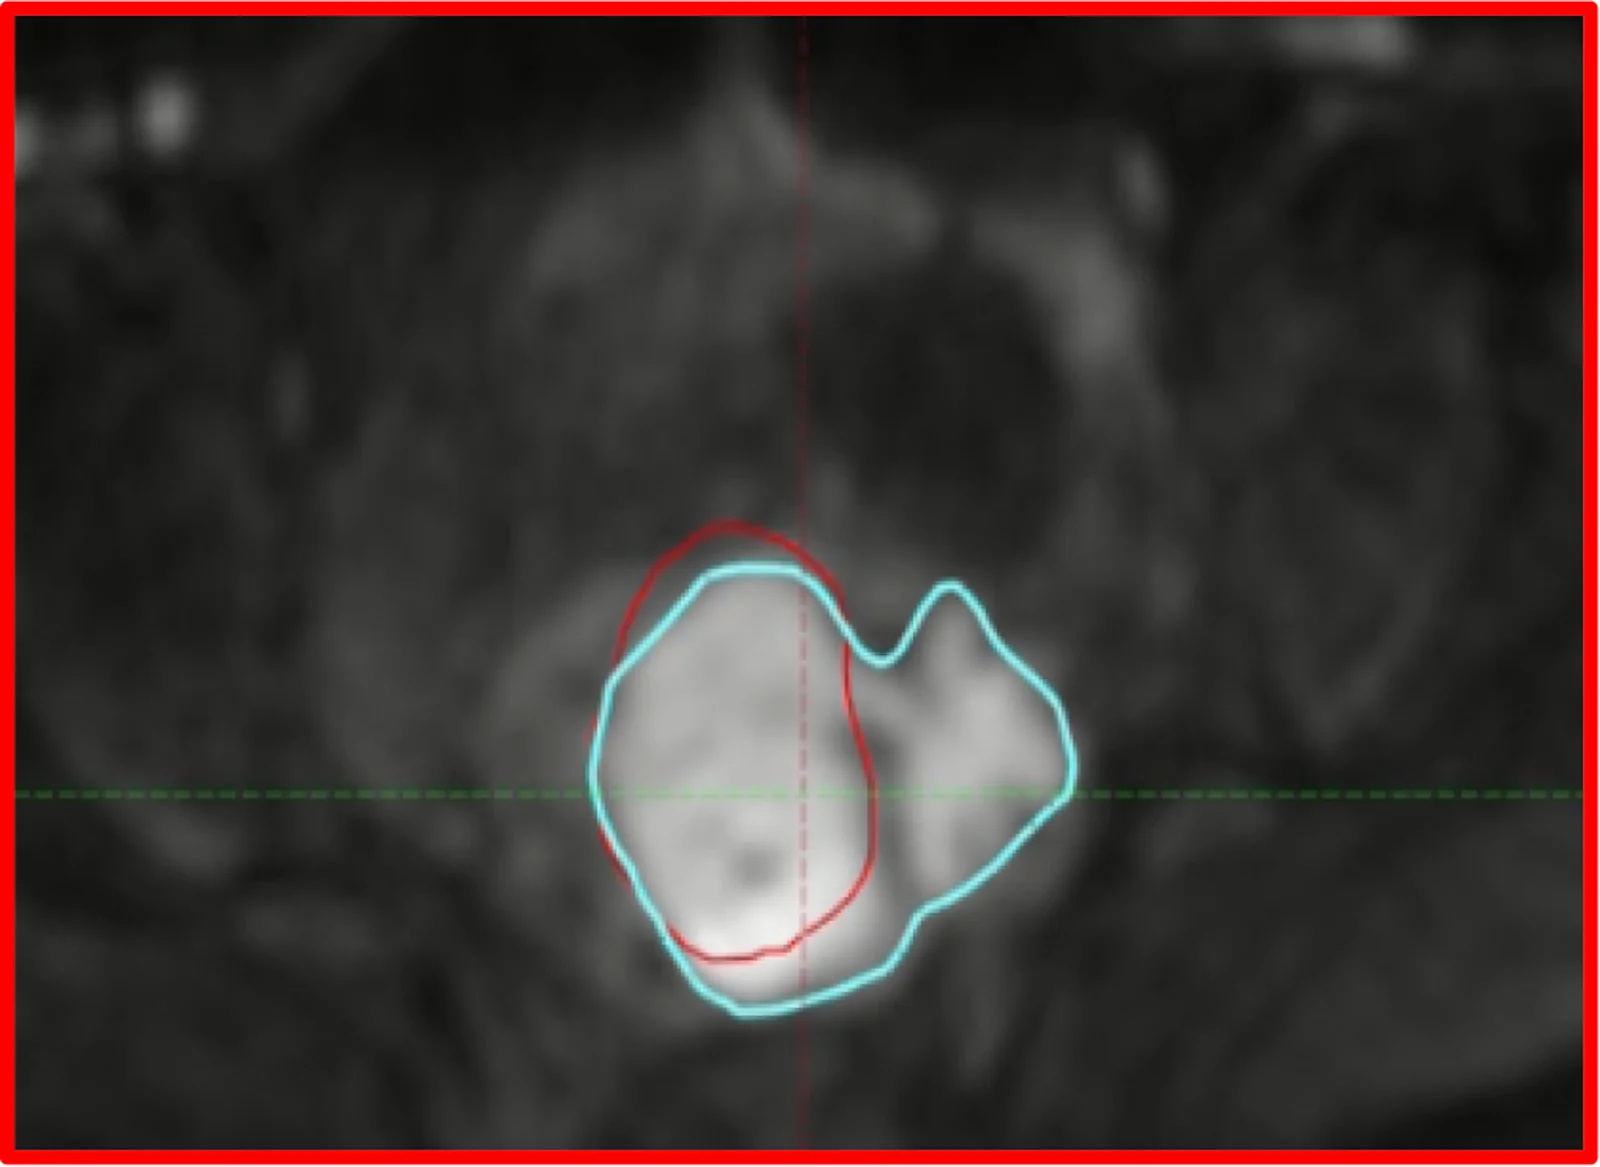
a. Figure depicting the GTV at pre_EBRT, GTV_T2 in red outline with an adjacent hypointense mass in left paracervical region. (For interpretation of the references to colour in this figure legend, the reader is referred to the web version of this article.) b. Figure showing the GTV at pre_EBRT; superimposed GTV_T2 in red outline and GTV_T2/DWI in cyan outline with high diffusion restriction area in left paracervical region corresponding to the hypointense T2 regions. (For interpretation of the references to colour in this figure legend, the reader is referred to the web version of this article.)
